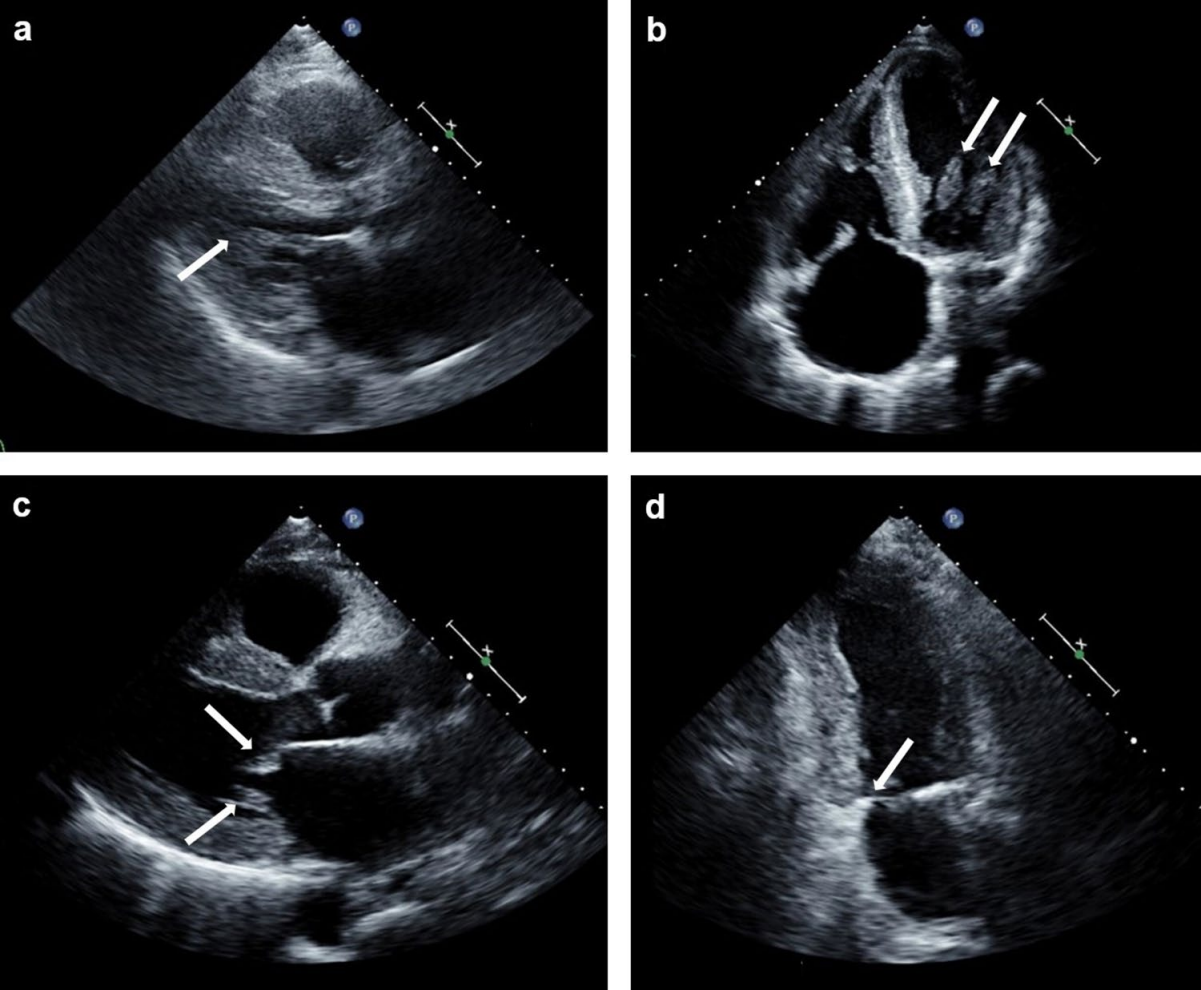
Features of mitral valve involvement in three men with cardiac transthyretin amyloidosis. a and b Thickened papillary muscles on a parasternal long-axis view (a) and an apical 4-chamber view (b), c parasternal long-axis view showing mitral leaflets thickening, and d apical 4 chambers view showing an almost hidden posterior mitral leaflet
